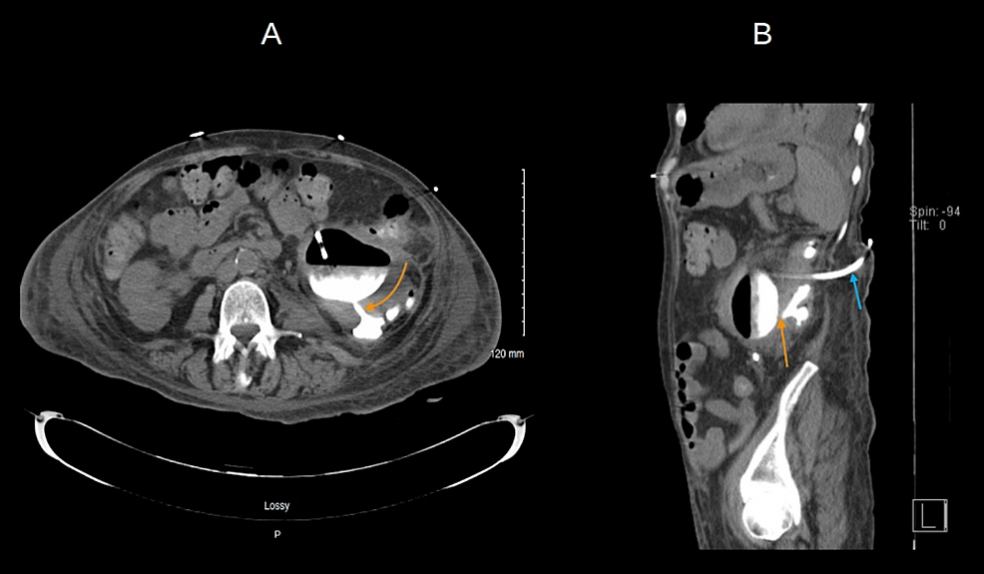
Axial and sagittal views of CT abdomen/pelvis delineating the colo-renal fistula tract A: Axial view of CT abdomen/pelvis demonstrating the renal pelvis with air and contrast. The arrow delineates the colo-renal fistula tract and below is the contrast opacifying the colon. B: Sagittal view of CT abdomen/pelvis demonstrating the nephrostomy tube tract (blue arrow), renal pelvis with air, and contrast opacifying the colon (indicated by the orange arrow).
